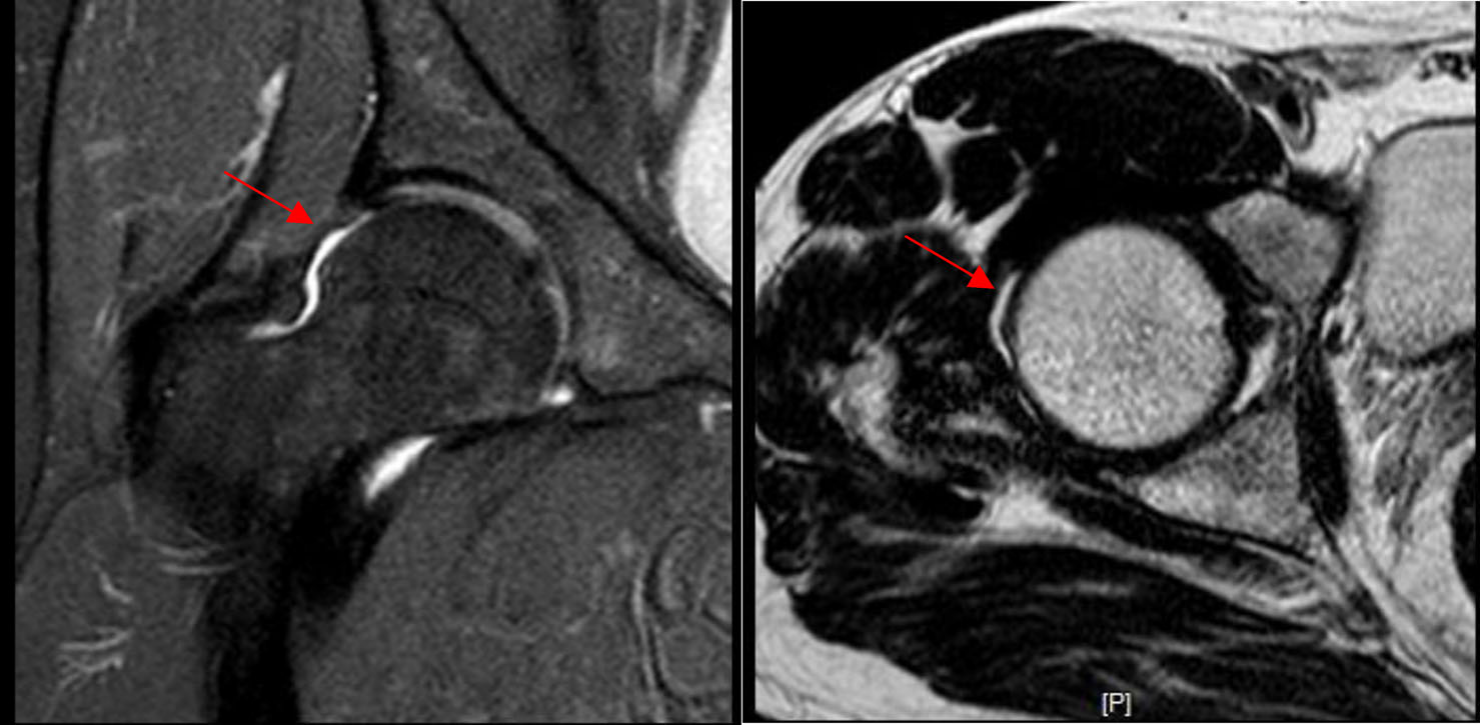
MRI shows 6 months after hip arthroscopy, the capsule has healed (red arrow).
